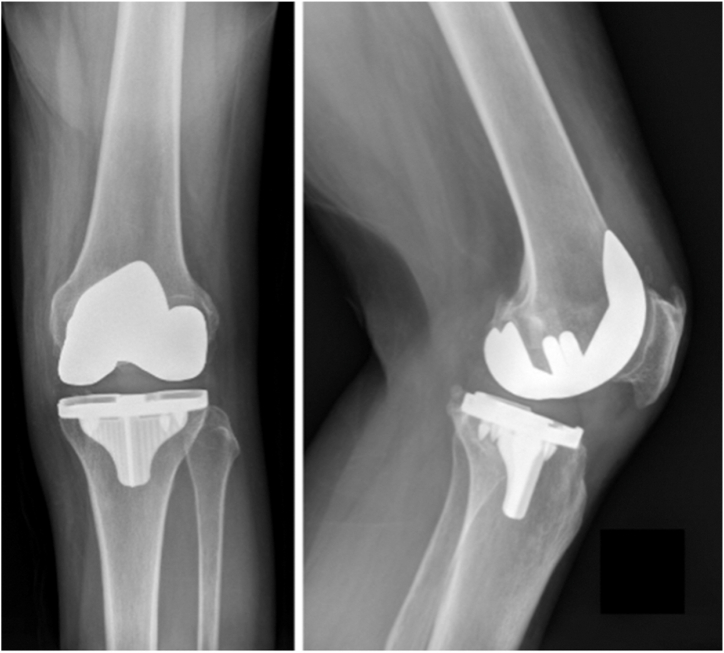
Anterior-posterior and lateral X-rays of the left TKA revision 18 months postoperatively.
